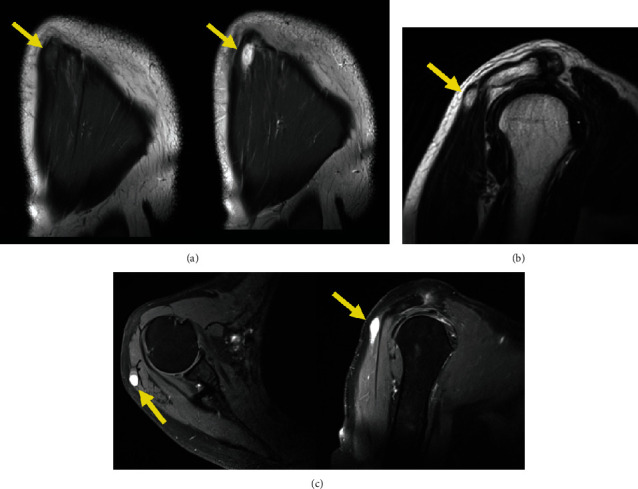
MRI images of the right shoulder. (a) Coronal images showing a nodular formation in the deltoid muscle (yellow arrows). The lesion is hypointense in T1 (left picture), but with strong enhancement with contrast (right picture). (b) Sagittal T2 image with turbo spin echo before contrast. The lesion appears as a hyperintense nodule (yellow arrow). (c) Axial (left) and sagittal (right) T1 images with fat suppression after contrast show a hyperintense well-limited lesion in the posterior deltoid (yellow arrows).
